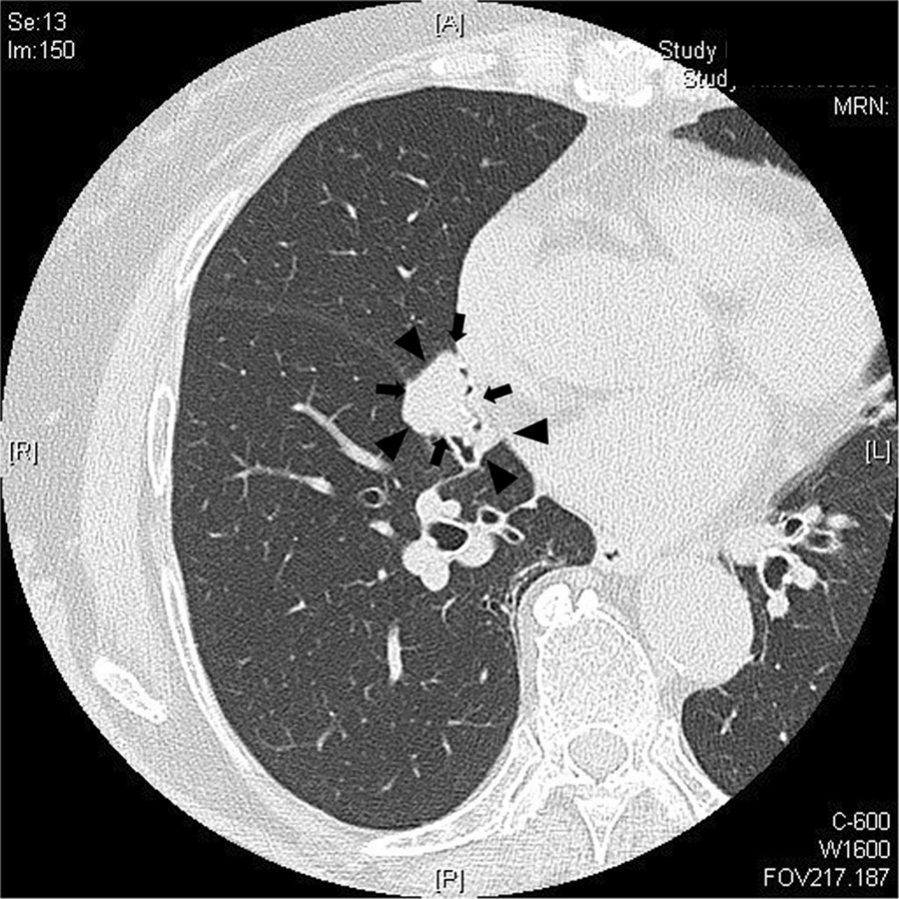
Chest CT showed a solid 2.5 × 2.0-cm nodule in the right middle lobe. Arrows indicate tumors and arrowheads indicate hypoplastic lungs. The right middle lobe was very small and was a hypoplastic lung. Most of the right middle lobe was occupied by tumor
